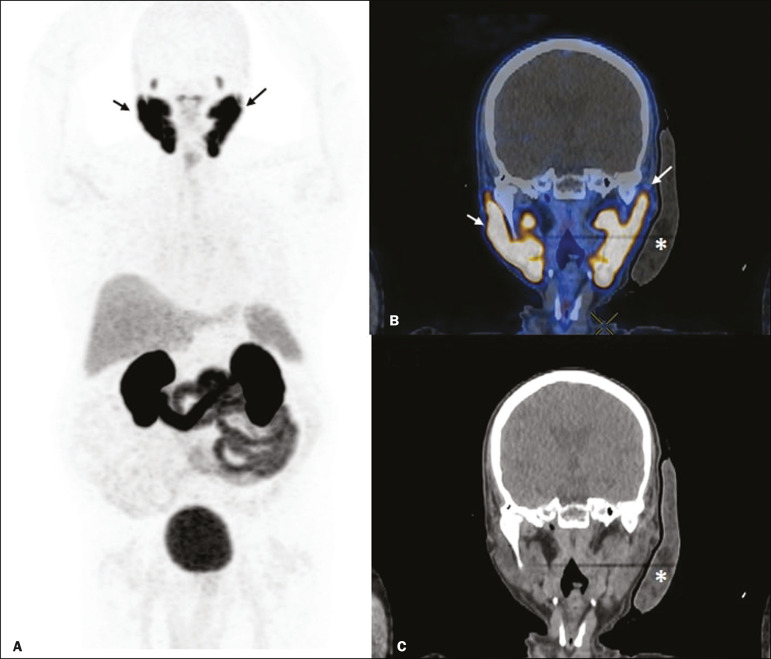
Maximum intensity projection PET (A) and coronal fused PET/CT (B) demonstrating physiologically accentuated uptake of 68Ga-PSMA by salivary glands (arrows) at t1. Coronal fused PET/CT (B) and CT (C) showing the position of the external cooling device (asterisks).
